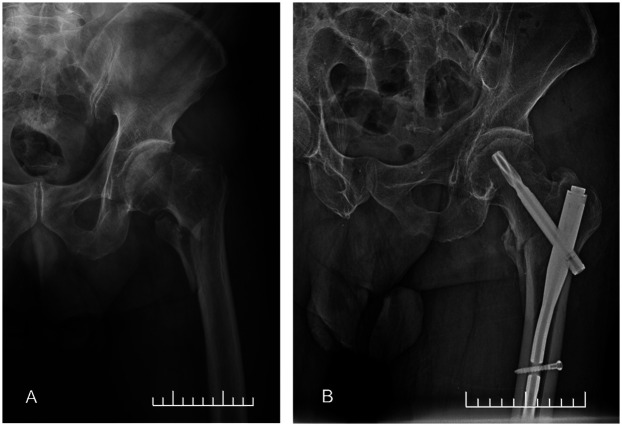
Internal fixation of intertrochanteric fracture. (A) Preoperative frontal x-ray. (B) Postoperative frontal x-ray.
